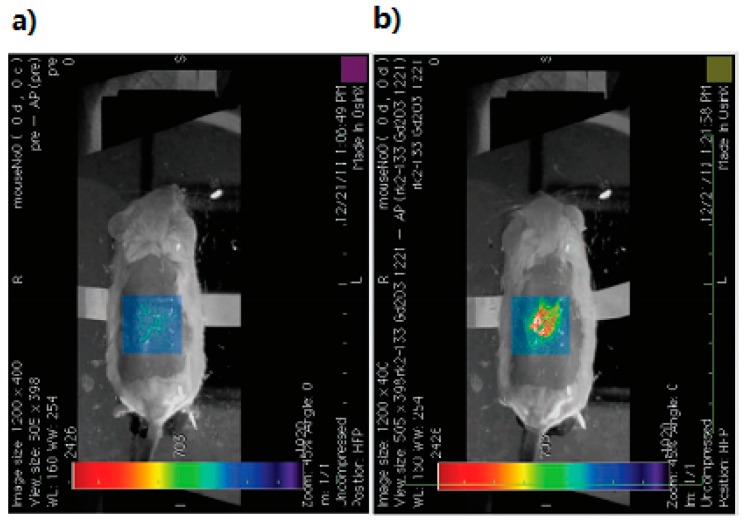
PAT-MRI images of tumor (a) before and (b) after injection of Gd_2_O_3_-DEG-gelatin. (Reprinted from reference [46]. Copyright with permission from © 2012 WILEY-VCH Verlag GmbH & Co. KGaA, Weinheim).
